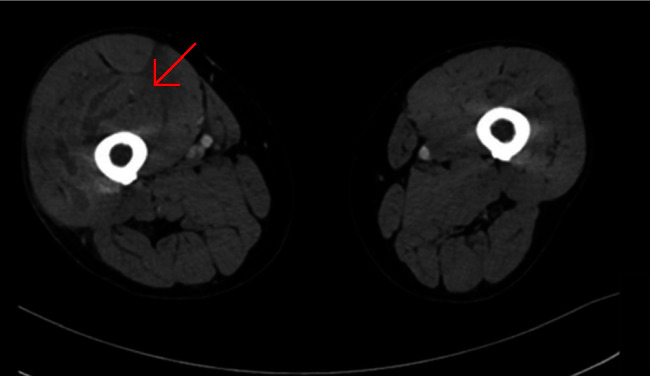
Axial view of a CT scan, demonstrating an increase in right thigh circumference.
